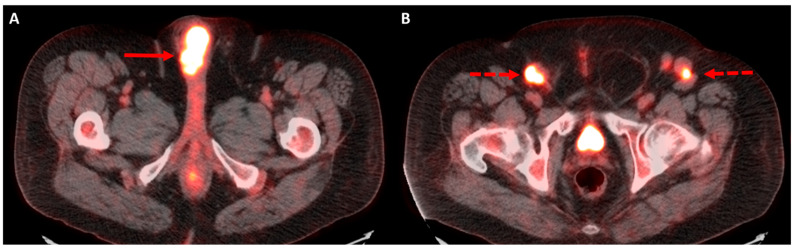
66-year-old male with history of penile cancer status post penectomy presents with suspected recurrence on clinical exam. Restaging FDG-PET/CT demonstrates an intensely hypermetabolic mass at the penectomy bed (A, solid arrow) and bilateral hypermetabolic inguinal lymph node metastases (B, dashed arrows).
